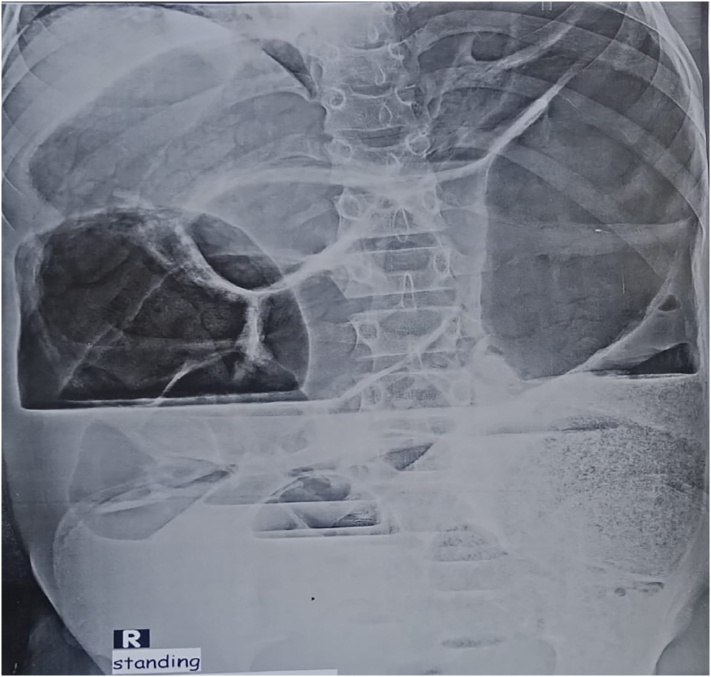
Plain erect abdominal X-ray: massive colonic distention with multiple fluid levels and ground-glass appearance suggesting fecal impaction at the distal part of the sigmoid colon.
